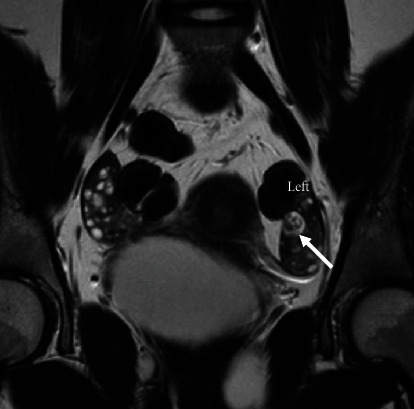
Repeat MRI abdomen/pelvis demonstrating 1.2 × 1.0 × 1.2 cm complex structure with macroscopic fat in the left ovary concerning for recurrent unilateral teratoma.
